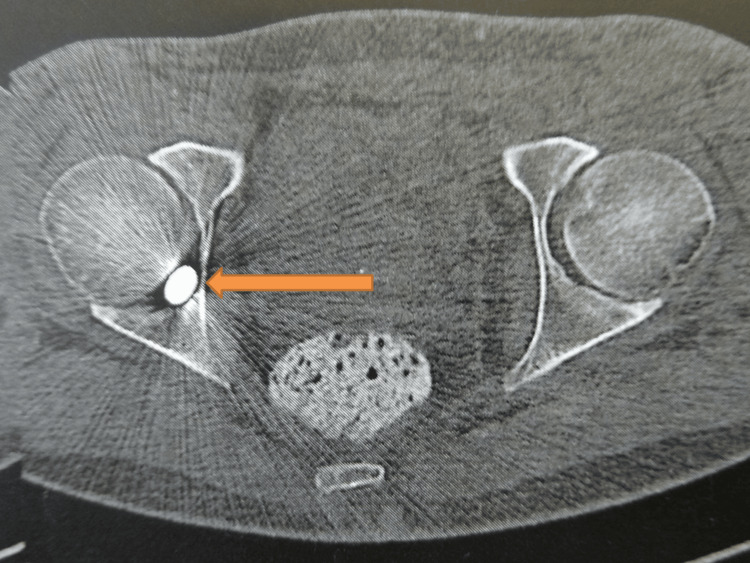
Axial section of the CT scan of the hips Arrow indicates the exact location of the bullet in the cotyloid fossa
